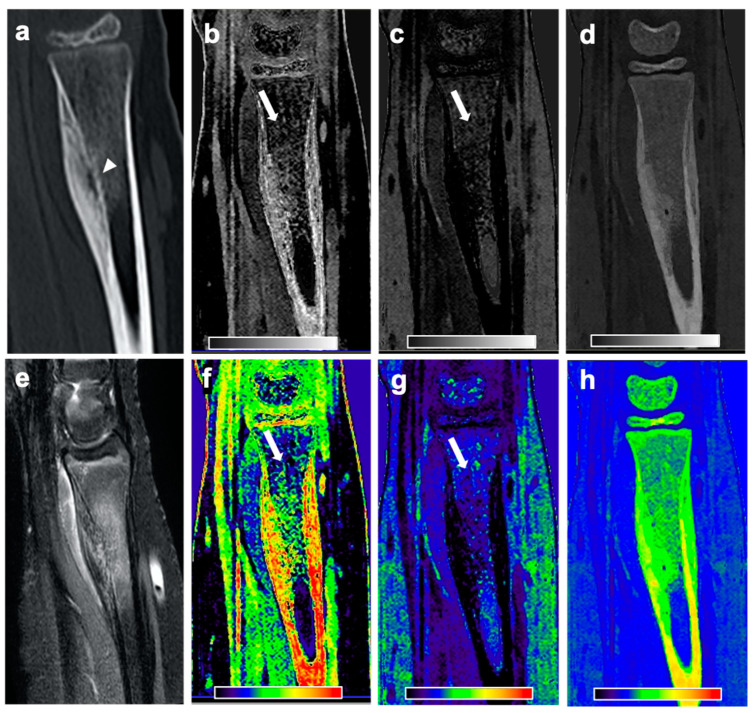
CT and MR images of a 12-year-old female patient with subperiosteal OO in the right distal radius. Sagittal CT reformation image (a) reveals the nidus in the right distal radius with surrounding sclerosis and a solid periosteal reaction (arrowhead). Material-specific volume fraction maps for red marrow/water (b), yellow marrow/fat (c), and hydroxyapatite (d), and respective color-coded overlays (f–h) reveal an increase in water-specific volume fraction in the red-marrow map (b,f; arrow) and a decrease in fat-specific volume fraction in the yellow-marrow map (c,g; arrow) in the right femoral neck, compared to the respective area on the other side. The corresponding sagittal STIR MR image confirms the presence of an edema-equivalent signal alteration in the bone marrow around the nidus (e).
